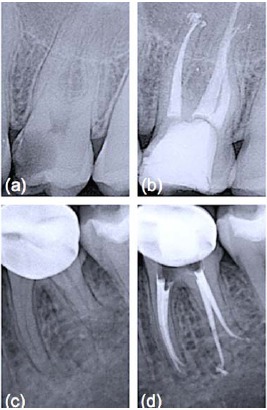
Periapical x-rays before and after root canal treatments. (a) Preoperative radiograph of upper left first molar (26); (b) One-year follow up radiograph of 26 obturated with vertically condensed gutta-percha and an epoxy-resin sealer; (c) Lower left first molar (36) before root canal treatment; (d) One-year follow up radiograph of 36 obturated with vertically condensed Resilon™ and RealSeal™ sealer. Note the similar radiopacity of the two filling materials (b and d).
